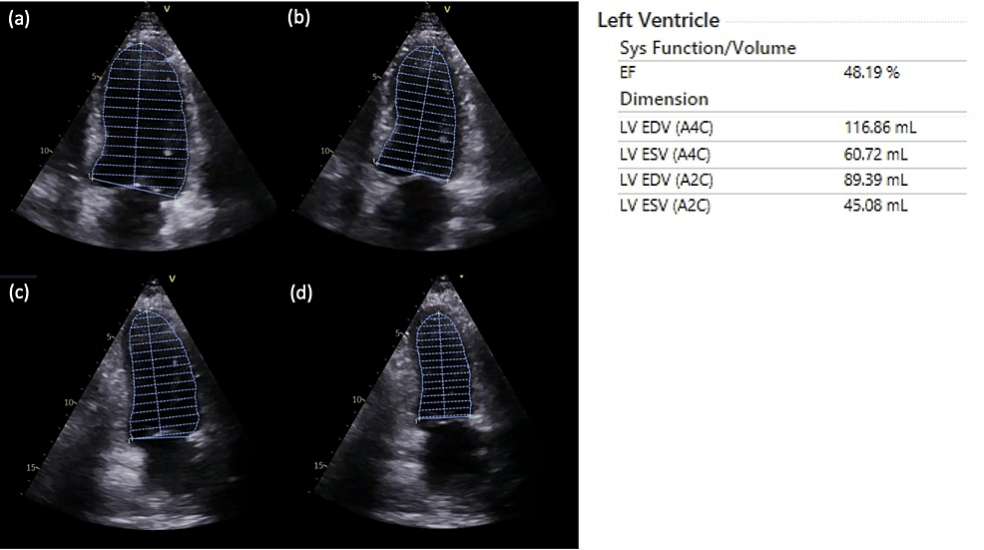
Quantification of left ventricular (LV) dimensions and ejection fraction (EF) with transthoracic echocardiography. (a) LV end-diastolic volume (EDV) in apical four-chamber (A4C) view; (b) LV end-systolic volume (ESV) in A4C view; (c) LV EDV in apical two-chamber (A2C) view; (d) LV ESV in A2C view.
